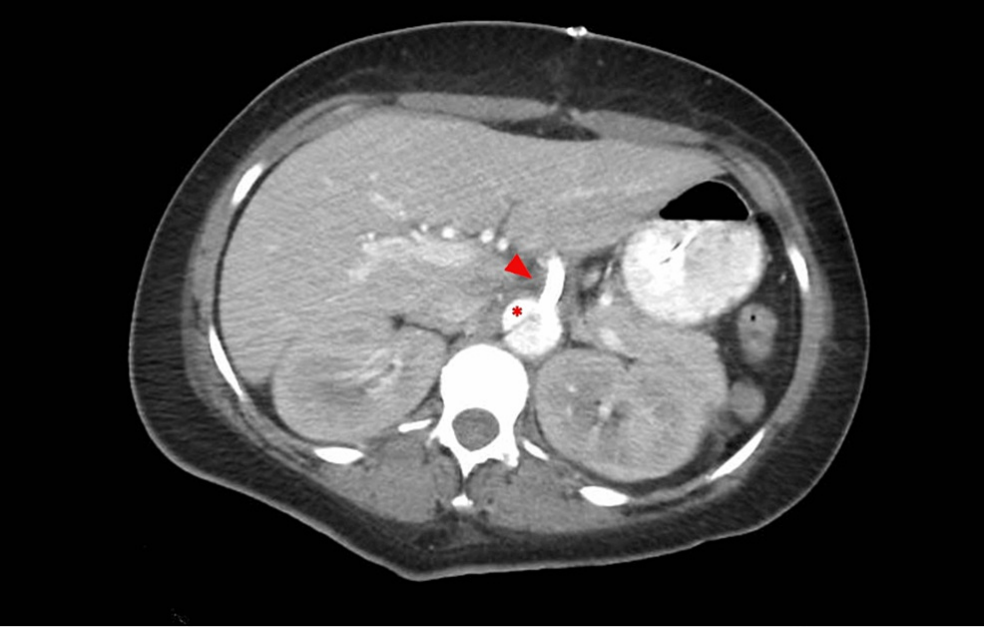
Postoperative computed tomography scan shows the patent celiac trunk stent. The red arrow indicates the celiac stent; * indicates true lumen
